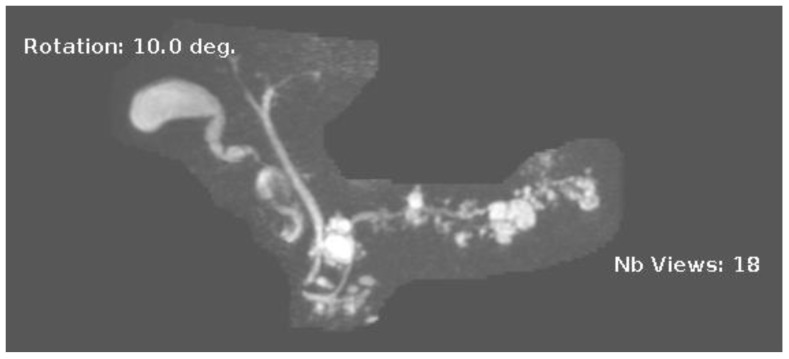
MRI image of side branch IPMN.
